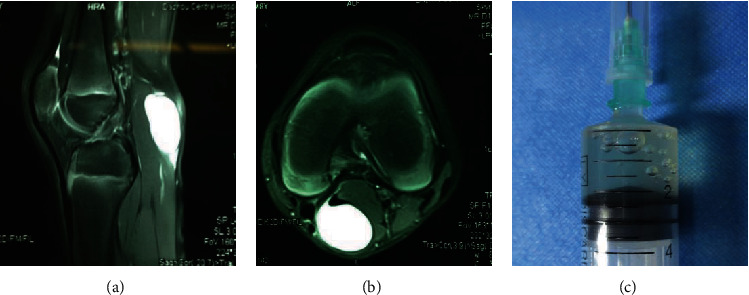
(a) T2-weighted sagittal scan and (b) T2-weighted axial scan of the right knee showing a cystic lesion between the semimembranous muscle and the medial head of the gastrocnemius. The popliteal cyst is filled with liquid. (c) Extraction fluid of popliteal cyst: it shows a colorless mucous liquid with little blood color.
